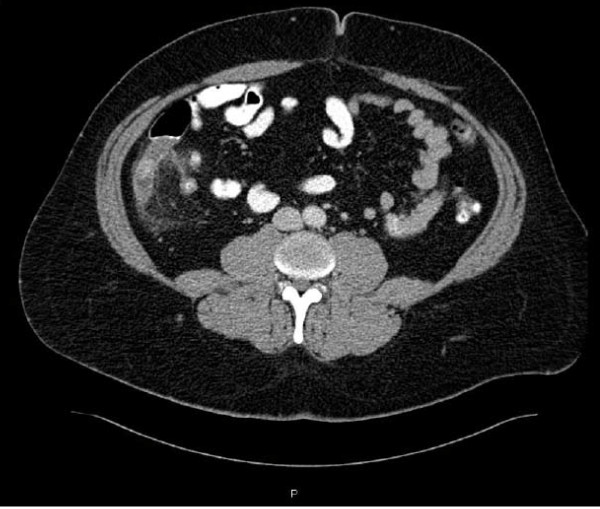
Computed Tomography image showing the enlarged and inflamed appendix.
